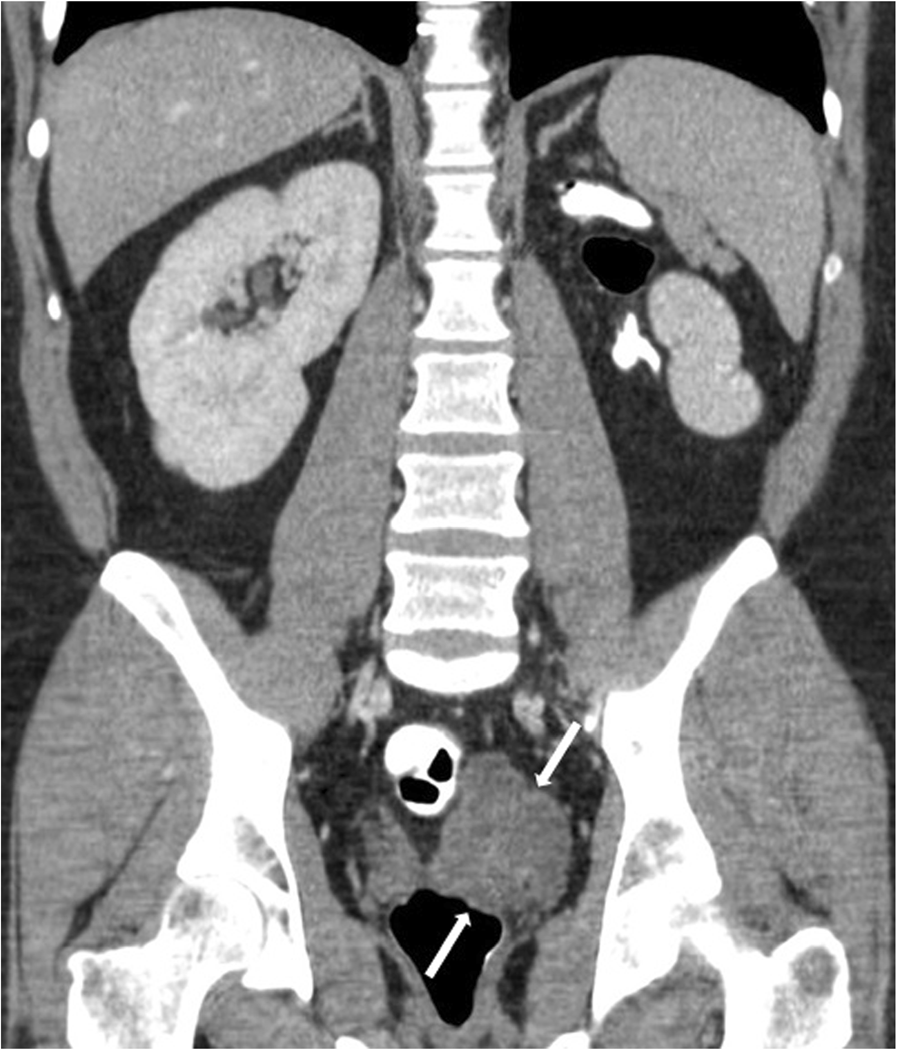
A 31-year-old male patient presenting with left groin pain. Coronal plane postcontrast CT image demonstrates left renal agenesis and left seminal vesicle cysts (arrows)
